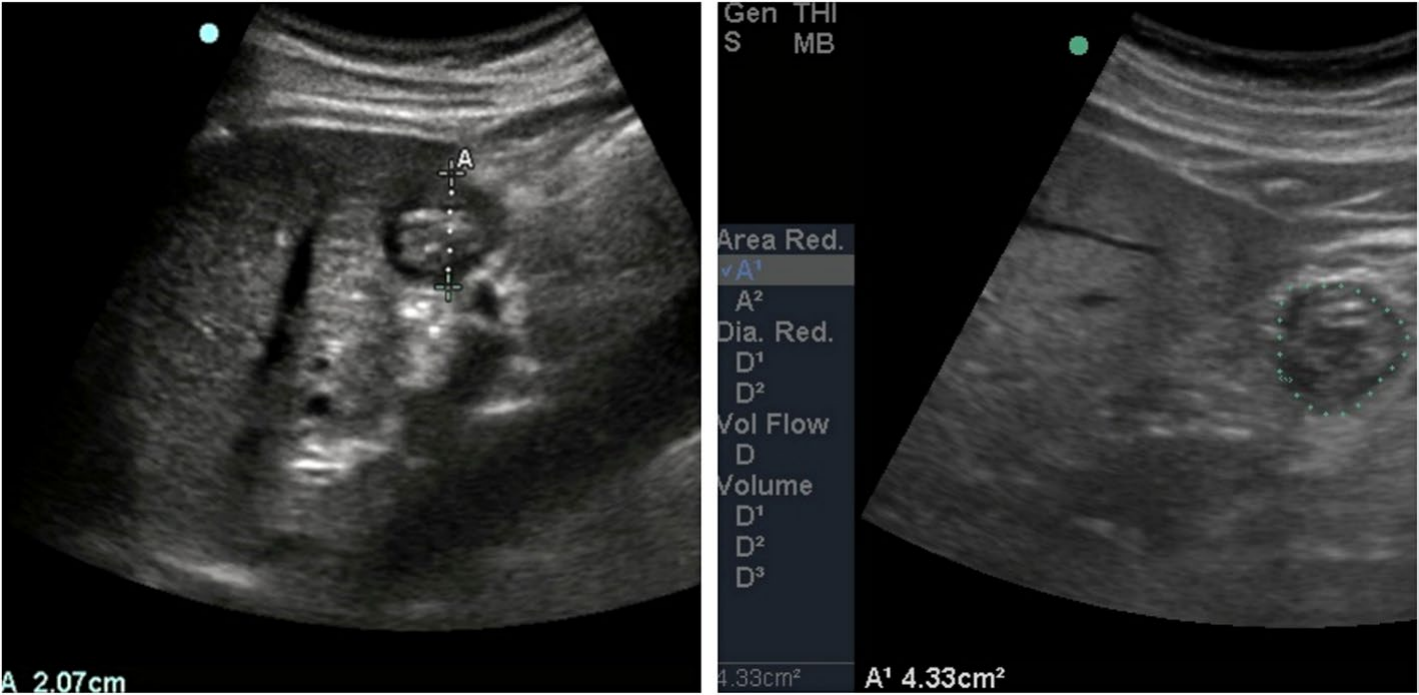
Scan and calculate the area of gastric antrum. Section of gastric antrum was taken by ultrasound, and then use Simpson 's integral method, trace along the edge of the gastric antrum, then utilizing ultrasonic data packet to scan and calculate the area of gastric antrum, we applied the following formula [6]: GRV (ml) = 27.0 + 14.6 × right-lateral CSA − 1.28 × age, to evaluate gastric volume
